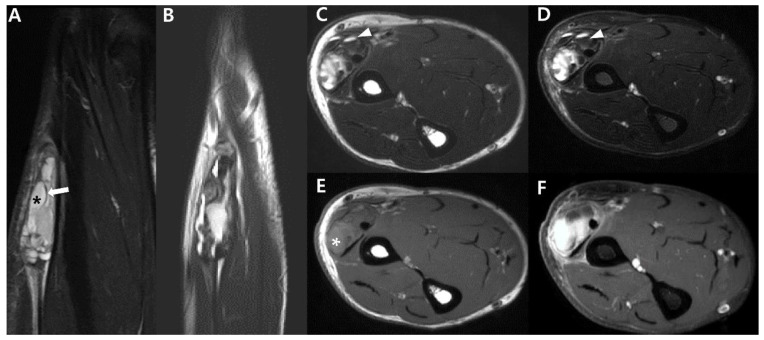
Aggressive features on MRI for large heterogenous mass of synovial sarcoma of 61-year-old-man (monophasic type): (A) Coronal T2-weighted, fat-suppressed image shows about 1.5 × 2.3 × 7.0 cm sized multilobulated mass with heterogenous signal intensity of Lt forearm. The mass shows presence of T2 hyperintense areas (*) with intervening T2 hypointense septa (arrow), called ‘bowl of grapes’. (B) Sagittal T2-weighted image also shows heterogenous intensity. (C,D) Axial T2-weighted images without and with fat suppression show well-circumscribed heterogenous signal intensity mass with fluid–fluid level (arrowheads), suggesting presence of hemorrhage. (E) Axial T1-weighted image shows iso to high (*) signal intensity compared to adjacent muscle. High signal intensity region suggests hemorrhage component. (F) Axial T1-weighted, post-contrast image shows heterogeneous enhancement.
